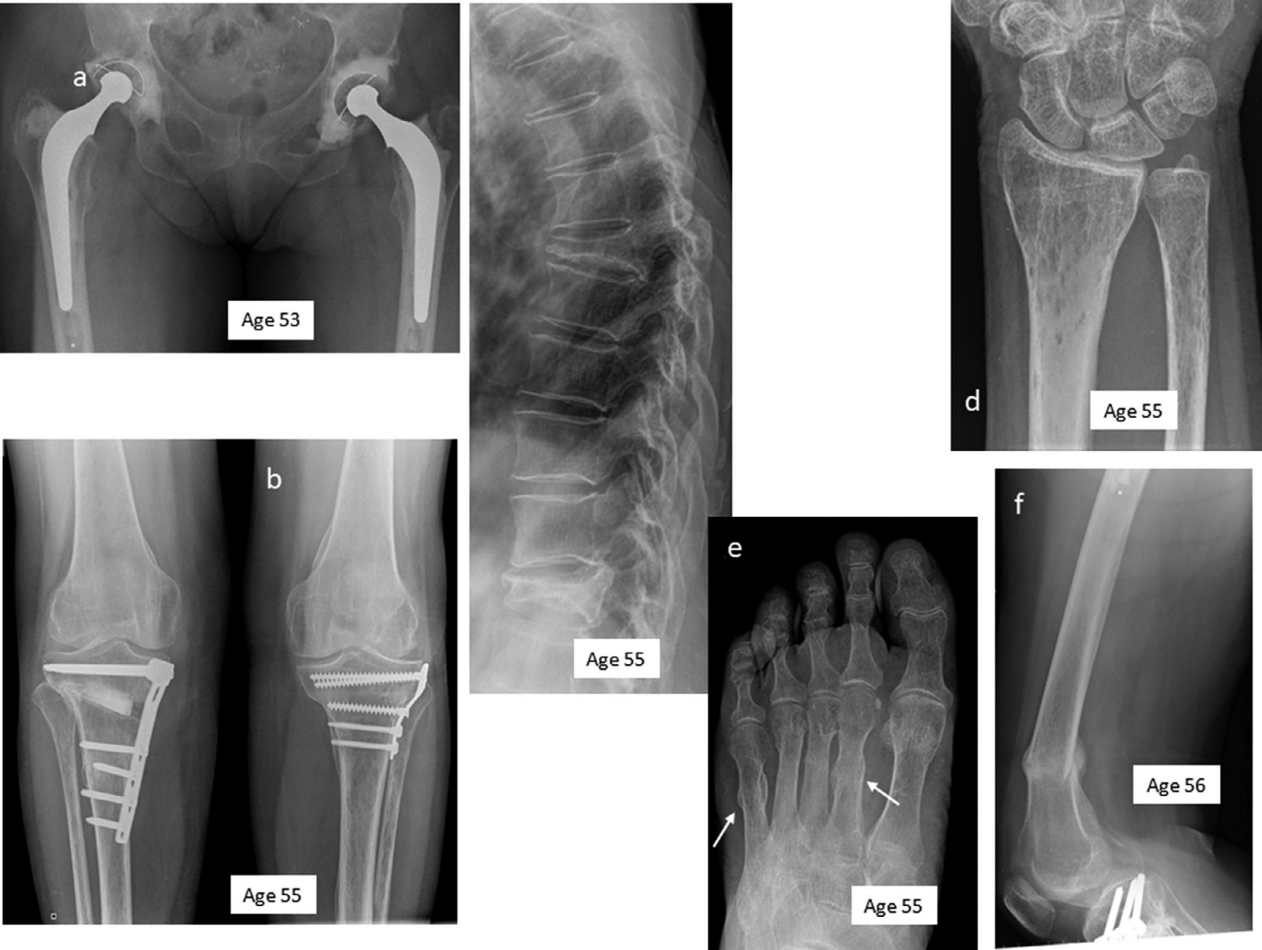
Selected radiographs from subject B. (*A*) Bilateral hip joint replacements after osteonecrosis of the hips, (*B*) bilateral osteotomy repairs for submetaphyseal tibial fractures, (*C*) vertebral fractures, (*D*) spotty osteoporosis of the distal radius and ulna, (*E*) healing metatarsal fractures (arrows), and (*F*) distal femoral fracture. The subject's age at the time of each radiograph is indicated.
